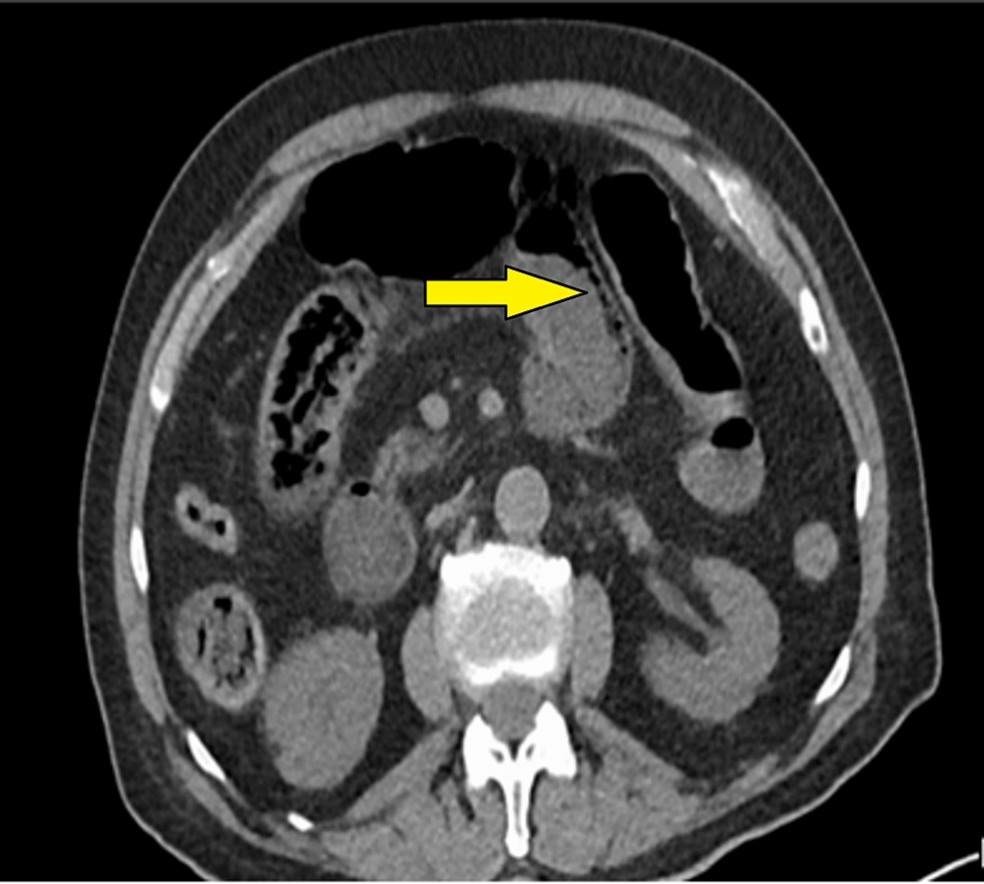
CT scan of the abdomen showing the presence of air within the small bowel segment (pneumatosis intestinalis).
